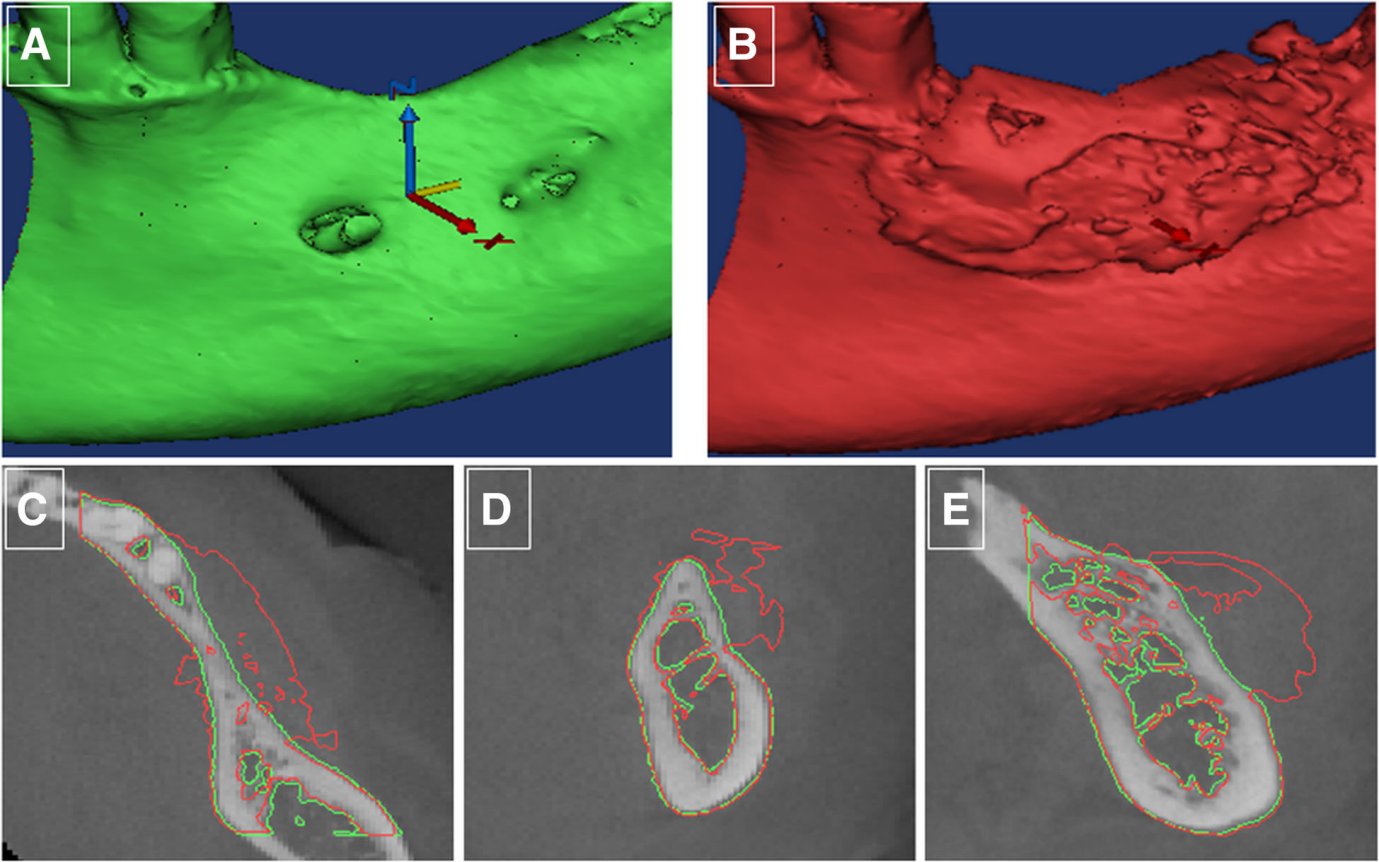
CBCT measurements. Overlapping of bone outline contours of superimposed models at T0 (before grafting, green) (a) and T1 (6 months after grafting, red) (b), achieved and viewed in axial (c), sagittal (d), and coronal (e) images of ridge before and after reconstruction
